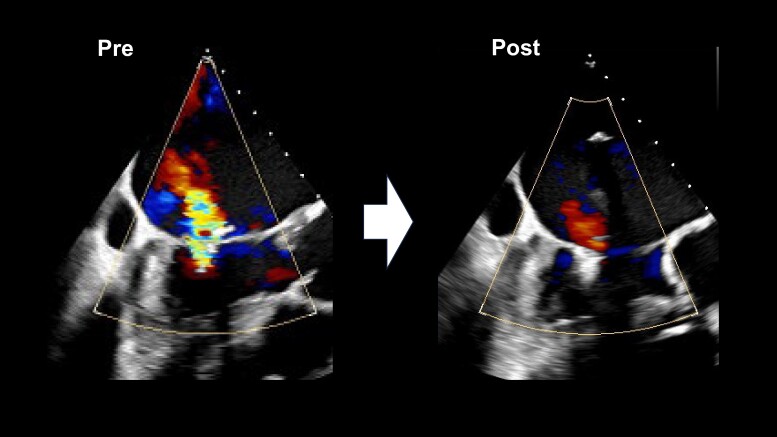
Intraoperative transoesophageal echocardiography (TOE) images before (left) and after transcatheter edge-to-edge repair (right) with improved mitral regurgitation.
